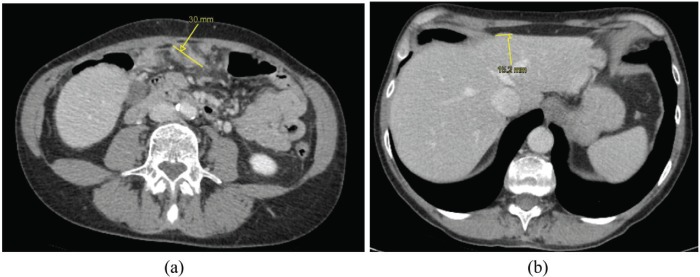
CT scan of the chest, including part of the superior abdomen, performed to complete staging after 2 weeks from the first CT scan, shows a dimensional and numerical reduction of peritoneal lesions [measures shown by yellow callipers in (a) and in (b)], as well as the resolution of perihepatic and peri-splenic ascites.
